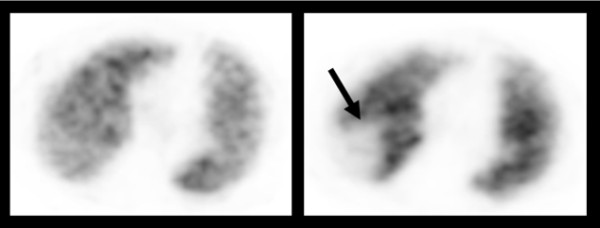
Perfusion defect detected by V/Q (SPECT reconstruction). Regular ventilation left, perfusion defect right (arrow).
